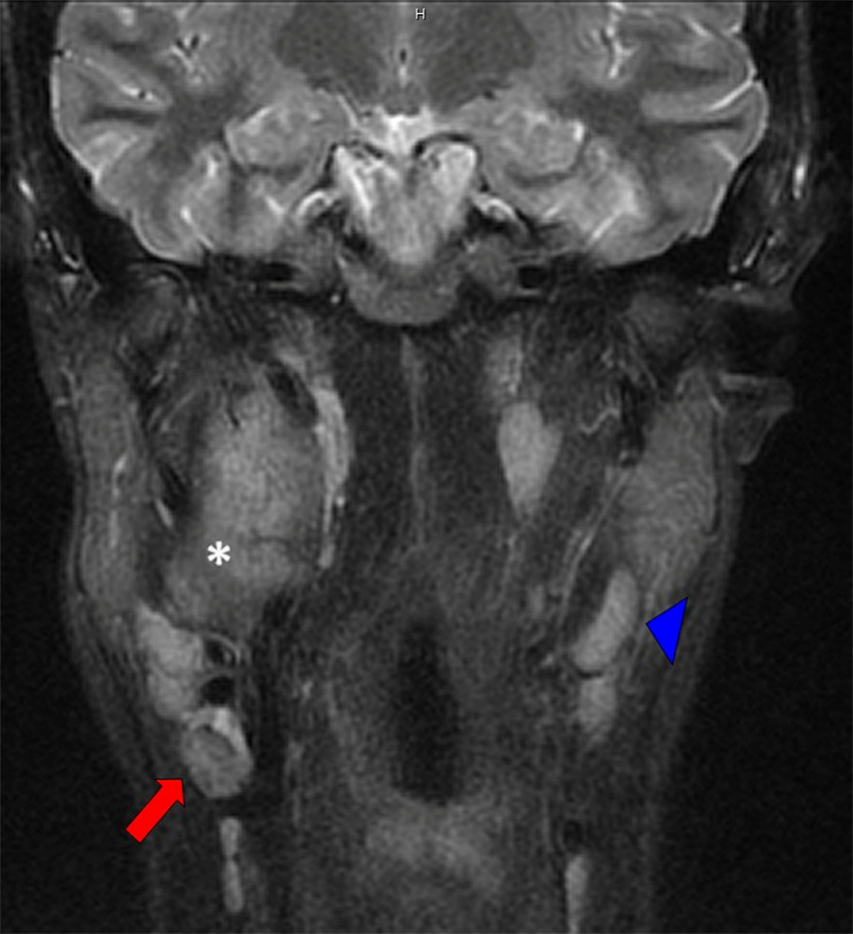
Coronal MRI short tau inversion-recovery sequence (STIR) demonstrating a right-sided carotid space mass of intermediate low signal (white asterisk) with an abnormal lymph node (red arrow) showing signal intensity similar to the mass. A contralateral normal lymph node is shown for comparison (blue arrowhead).
